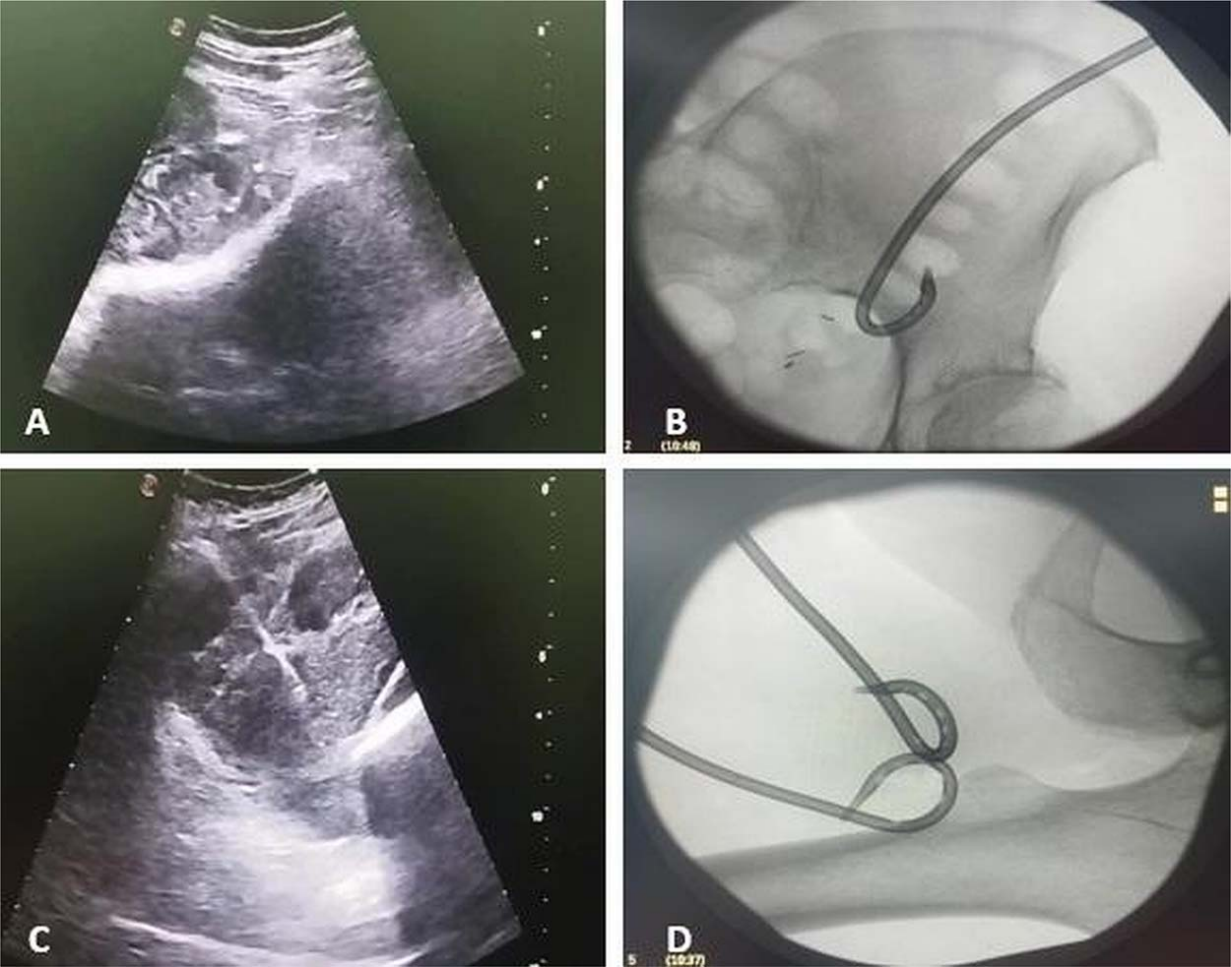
Ultrasound and fluoroscopy show multi-access percutaneous drainage with one retroperitoneal and two femoral catheters. (A) Ultrasound-guided retroperitoneal puncture into the psoas collection. (B) Fluoroscopic image demonstrating catheter placement in the psoas component. (C) Ultrasound-guided puncture of the thigh extension. (D) Fluoroscopic confirmation of one retroperitoneal and two femoral drains in situ.
